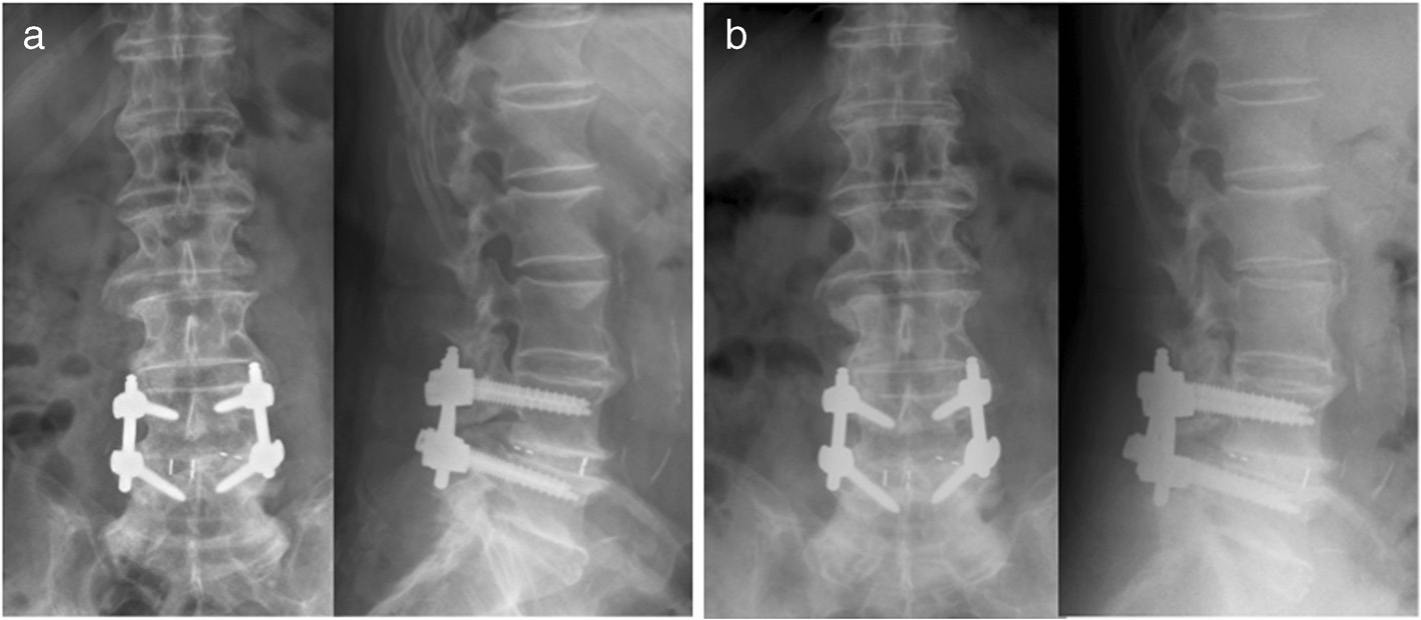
Post-operative a.p. and lateral conventional X-rays in an upright standing position at 3 months (a) and 1 year (b) postoperative follow-up
